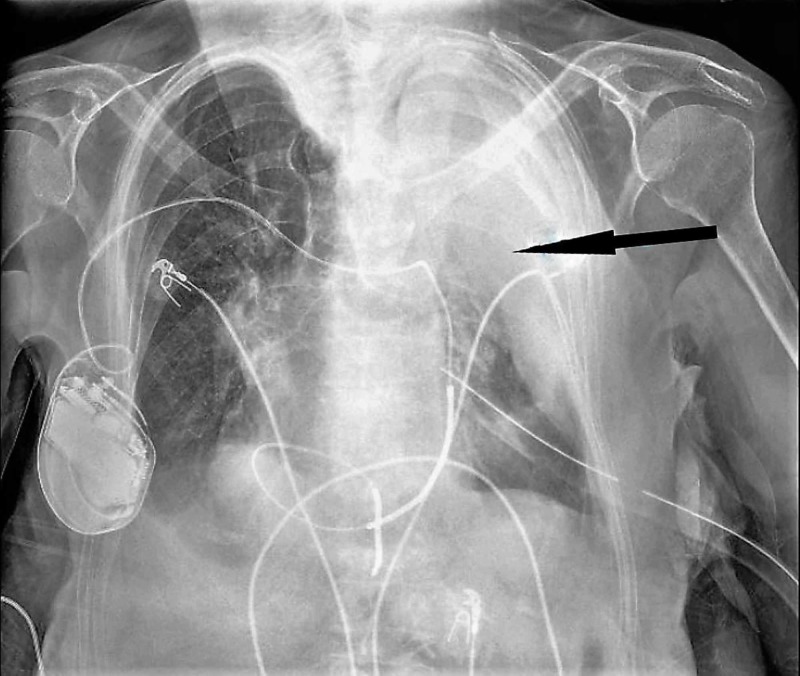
Chest X-ray after the placement of the chest tube
